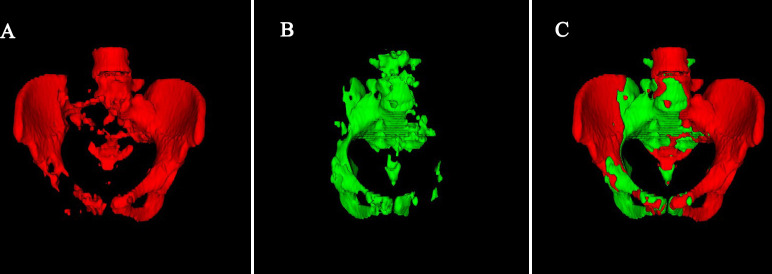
A case of BM_ACT_ and BM_TOT_ distribution. (A) Red indicates that bone SUV is lower than the average SUV of the patient’s individual mean SUV. (B) Green indicates that bone SUV is greater than or equal to the average SUV of the patient’s individual mean SUV. (C) Total volume of Pelvic bone.
